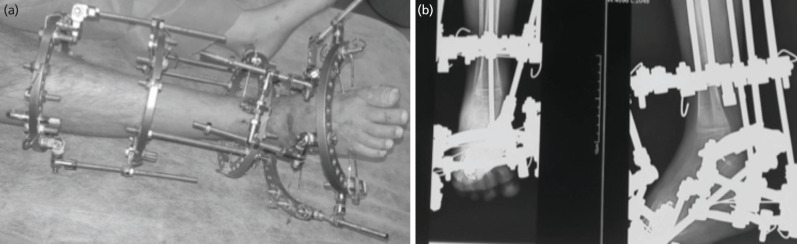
(a, b) Ilizarov Apparatus and post-operative radiographs.
